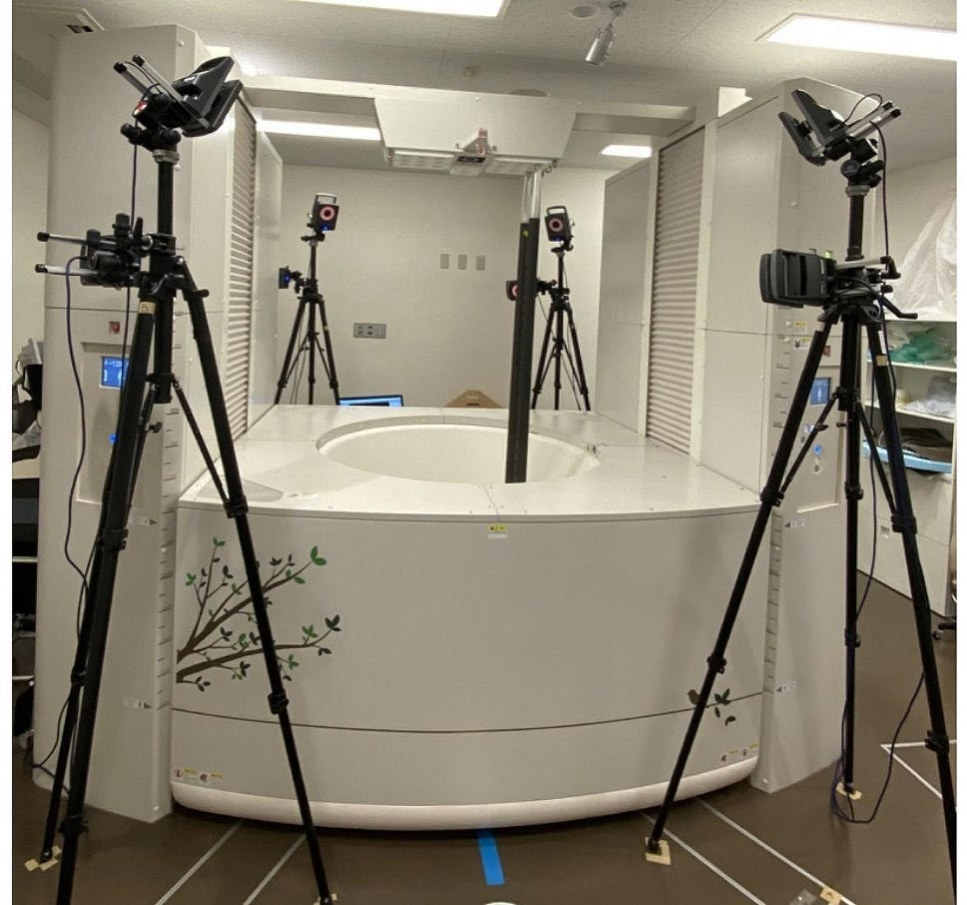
Upright computed tomography (CT) and optical motion capture cameras
